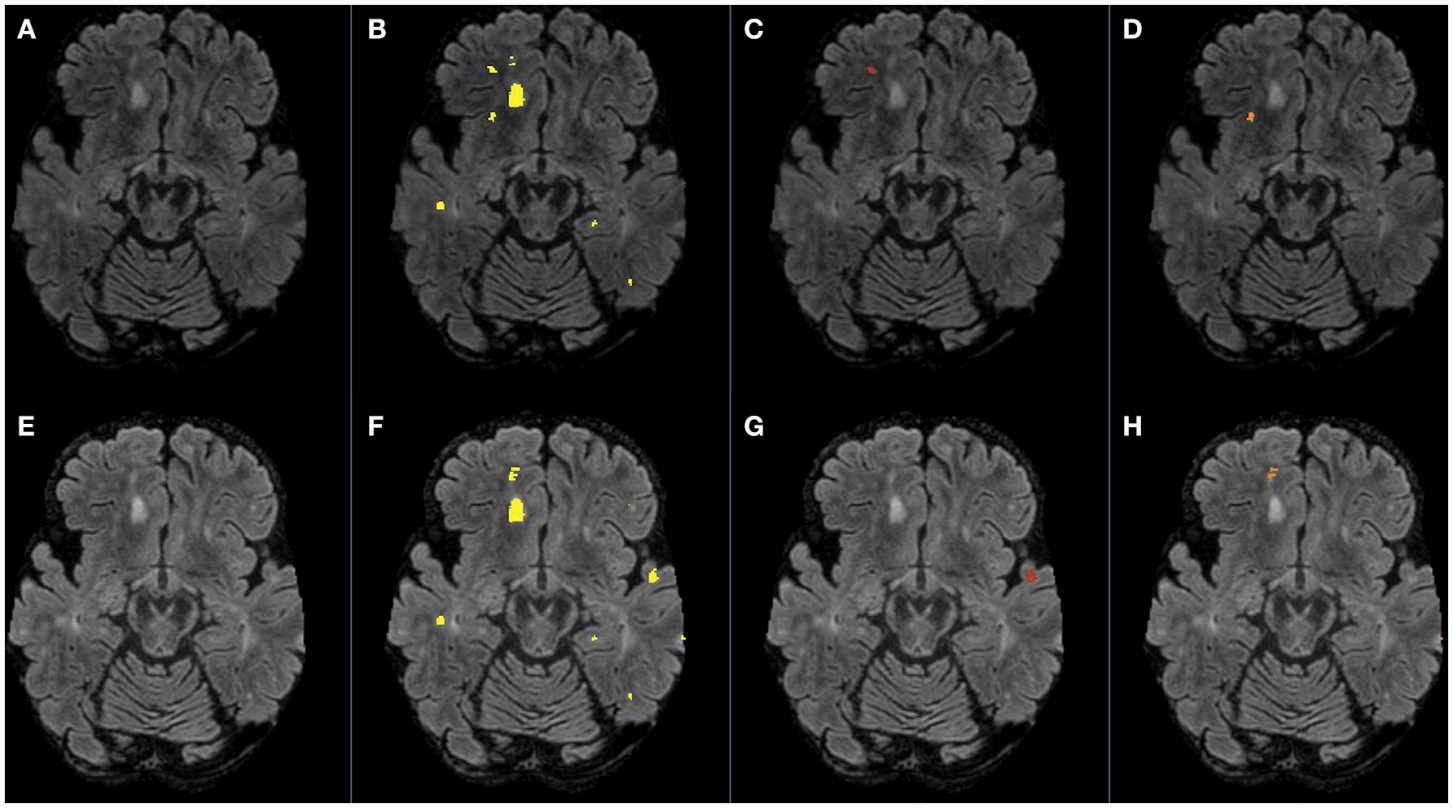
Bias corrected FLAIR images (A,E) followed by super-imposed lesion segmentations from: (B,F) the expert, (C) disappearing lesion, (D) shrinking lesion, (G) new lesion, and (H) enlarging lesion. The first row corresponds to time point 1 and the second row corresponds to time point 2.
